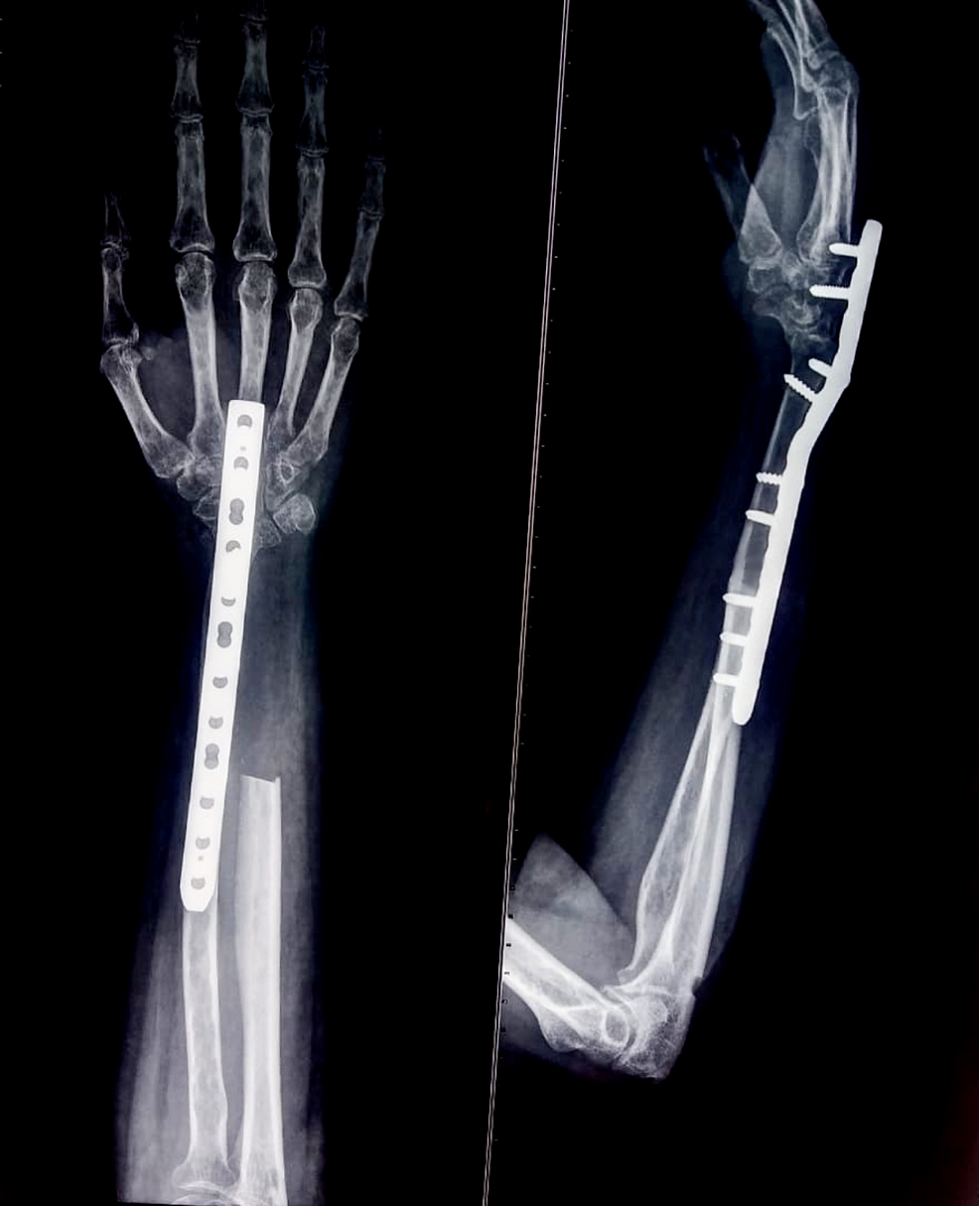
Two-year follow-up radiograph shows bony union at the radio-ulnar junction with no sign of recurrence.
